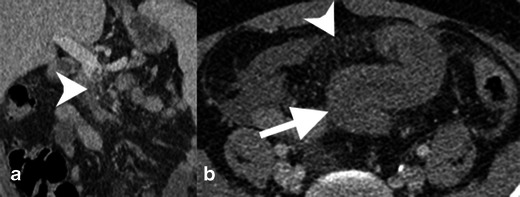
SMV thrombosis with mesenteric congestion. a Contrast-enhanced coronal reformatted CT of an occlusive thrombus in the SMV (*white arrowhead*) extending to the portal confluence. b Axial contrast-enhanced CT image demonstrates mesenteric congestion with engorged mesenteric veins and trace fluid (*white arrowhead*). The small bowel is thickened because of the venous stasis and probable ischaemia
